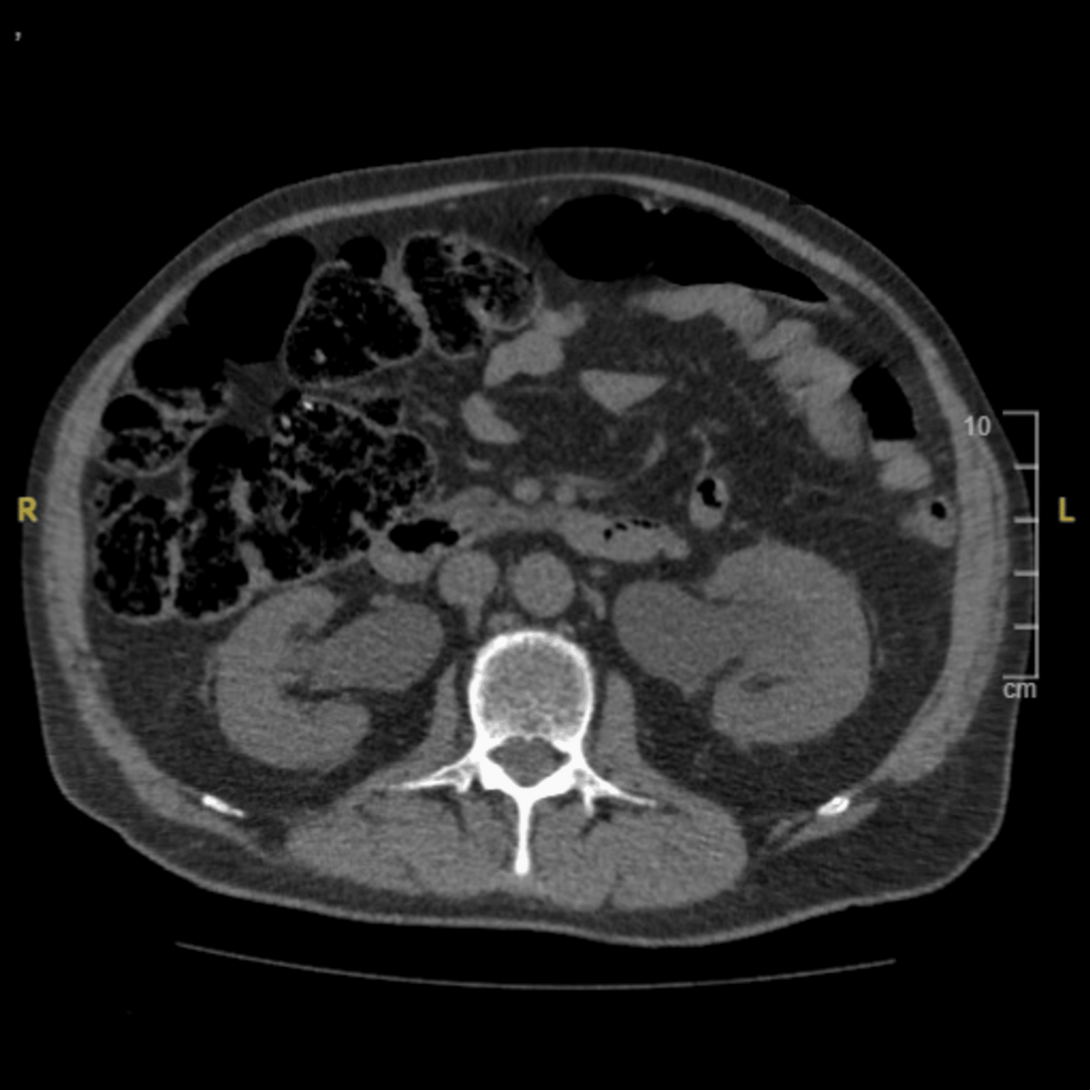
Axial view of abdominopelvic computed tomography showing a bilateral hydronephrosis.
